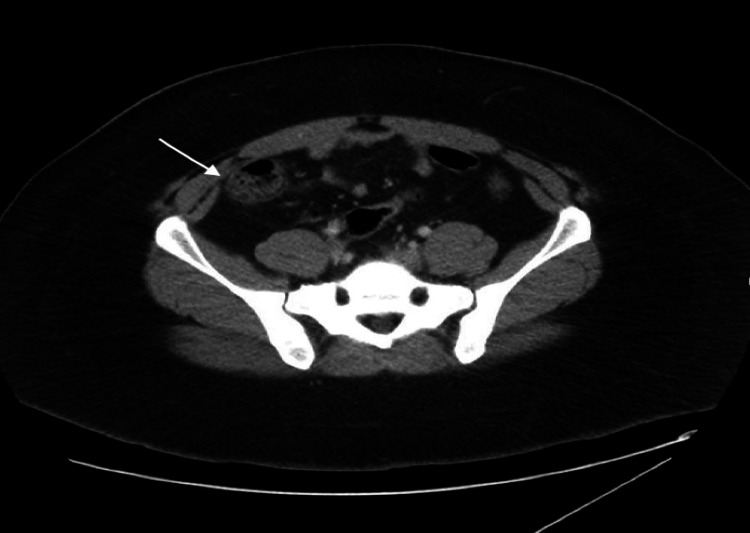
CT abdomen - axial view The arrow points towards the diverticula.
